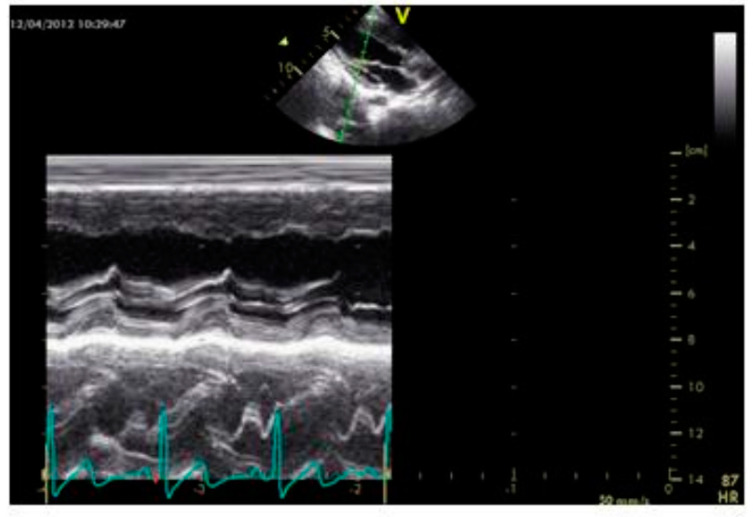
Showed M mode echocardiogram in β thalassemia major child.
